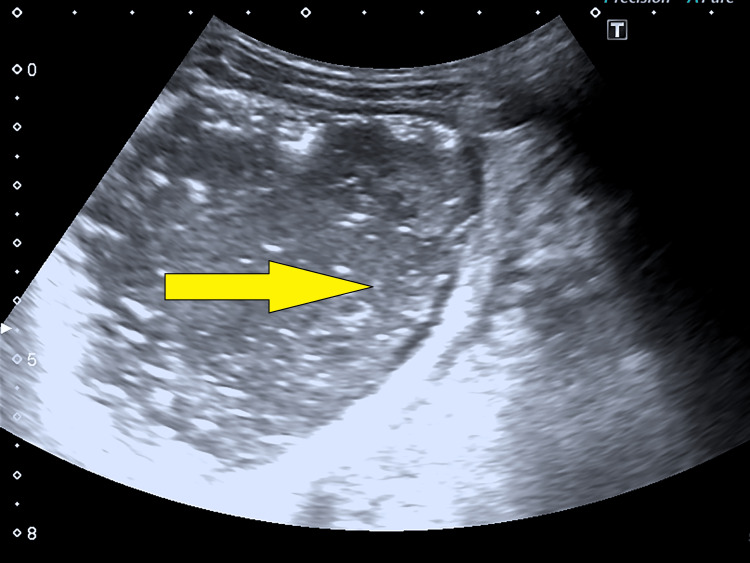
Abdominal ultrasonography showing free fluid in the abdomen with septations, suggestive of pyoperitoneum.
